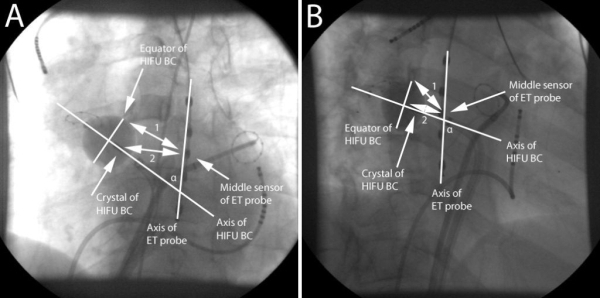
Analysis of distance from crystal and equator of HIFU balloon catheter to middle sensor of esophageal temperature probe and angle between axes of HIFU balloon catheter and esophageal temperature probe. A. LAO projection; B. RAO projection (HIFU: high-intensity focused ultrasound, balloon catheter: balloon catheter, LAO: left anterior oblique, 1: distance from equator of HIFU balloon catheter to middle sensor of ET probe, 2: distance from crystal of HIFU balloon catheter to middle sensor of ET probe, α: angle between axes of HIFU balloon catheter and ET probe, ET: esophageal temperature)
